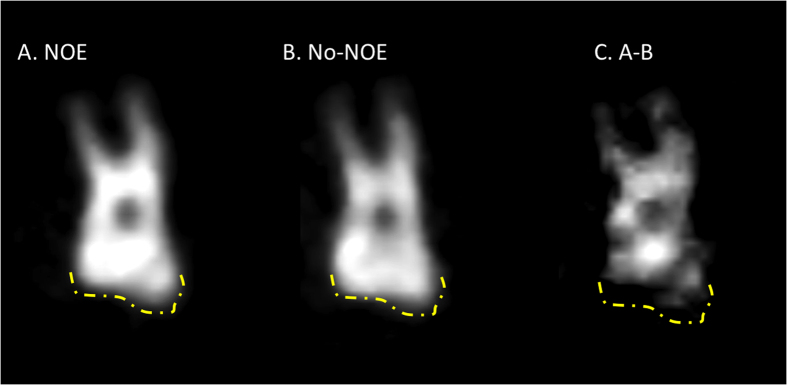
Selected slices of 3D ^31^P MR images of maxillary left second molar tooth obtained with the SWIFT sequence. (A) SWIFT image obtained with irradiation at the ^1^H frequency for 1.282 s, preceded by 64 dummy scans to reach steady state. (B) MR image of same slice obtained without ^1^H irradiation. (C) The subtraction of A and B shows a positive NOE in particular for signal coming from dentin. The yellow dash-dot line represents the contour of the enamel.
